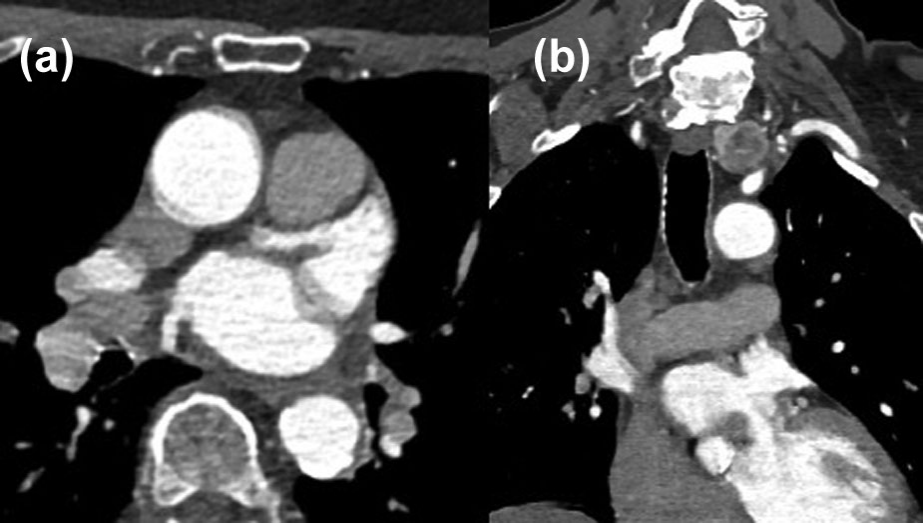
CTA imaging negative for LAA thrombus on axial (a) and coronal (b) sequences.
